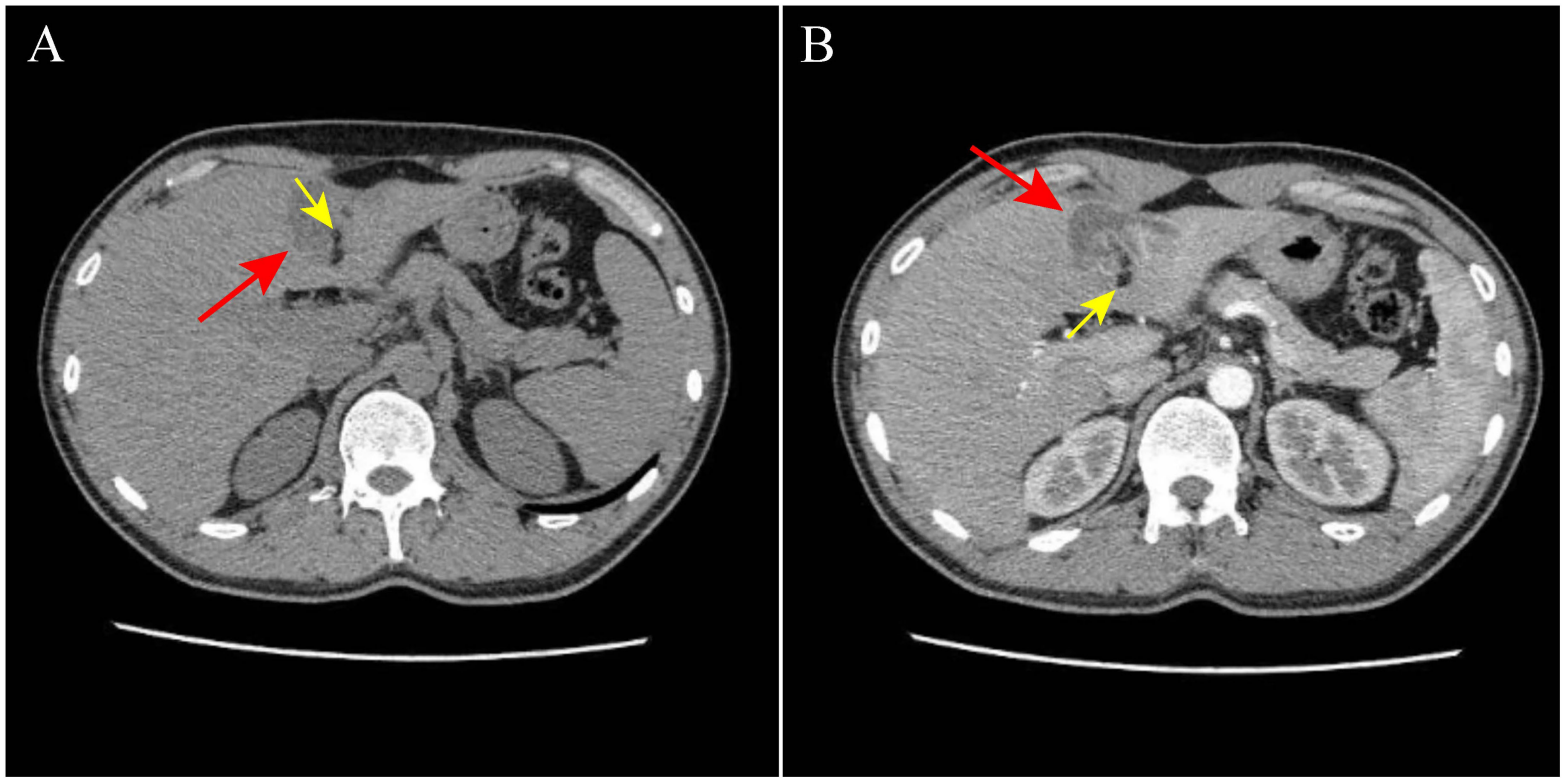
CT on May 27, 2024 (both parts A and B) showed that segmental enhancements and detached endocysts were in the liver lesions (both pointed by arrows).
